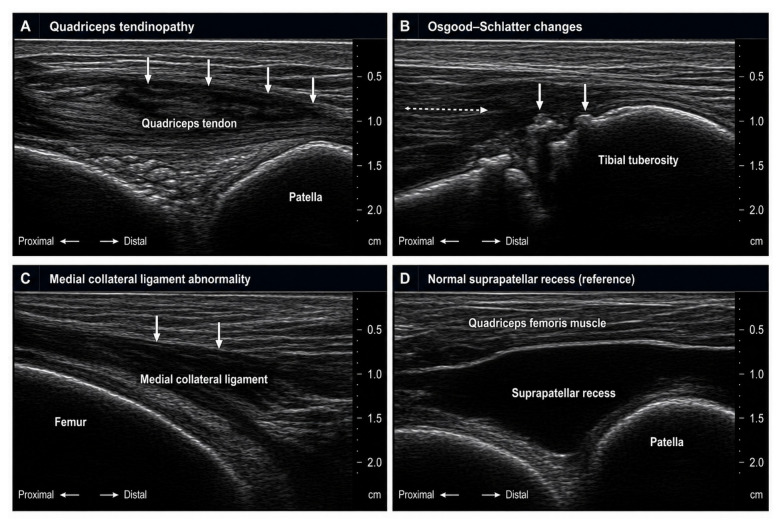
Additional representative ultrasonographic findings identified during the examination protocol. (A) Quadriceps tendinopathy demonstrating focal tendon thickening and hypoechogenicity (solid arrows). (B) Osgood–Schlatter changes involving the tibial tuberosity, with irregular ossification at the tibial tuberosity (solid arrows) and distal patellar tendon insertion (dashed arrow). (C) Medial collateral ligament abnormality demonstrating focal ligament thickening and altered echotexture (solid arrows). (D) Normal ultrasonographic appearance of the suprapatellar recess (reference image).
